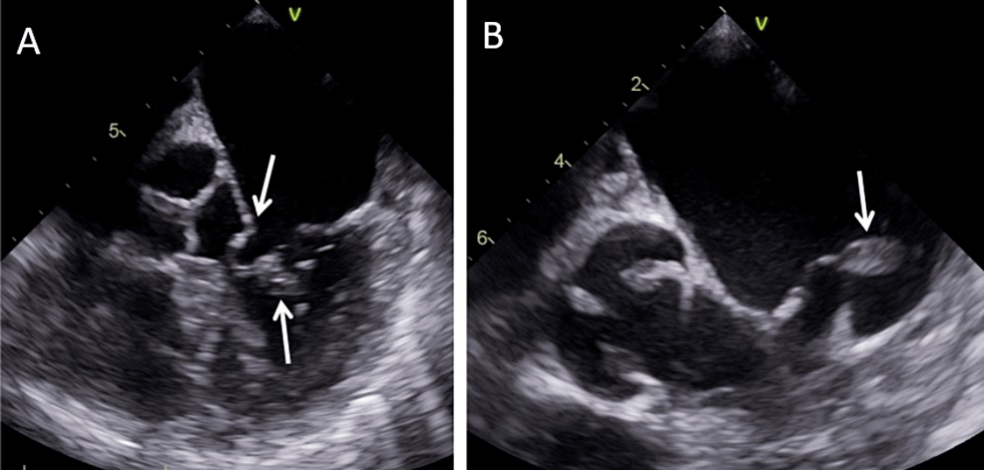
Comprehensive transesophageal echocardiogram Transesophageal echocardiogram (mid-esophageal 4-chamber view) demonstrating (A) flail motion of the anterior mitral valve leaflet (downward arrow) with adherent mass (upward arrow) and (B) ruptured anterolateral papillary muscle adherent to anterior mitral valve leaflet (arrow).
